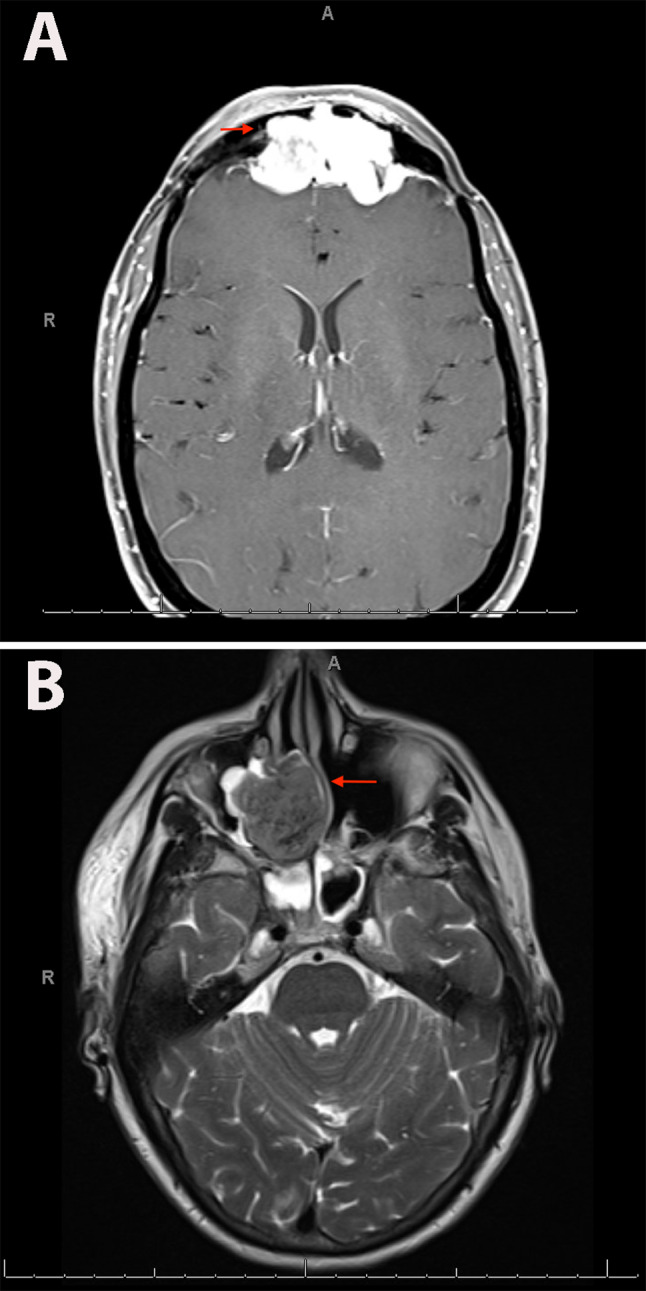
Biphenotypic sinonasal sarcoma, radiologic findings. A Patient one: MR orbit, face, and neck (T1-weighted, axial, post-contrast phase) shows a 5.6 cm enhancing mass centered in the frontal sinuses, with thickened, enhancing dura superior to the mass. B Patient two: MR brain (T2-weighted, axial) shows a 4.7 cm right paramedian sinonasal mass that obliterates the ethmoid air cells and the right nasal cavity and extends into the right maxillary sinus
